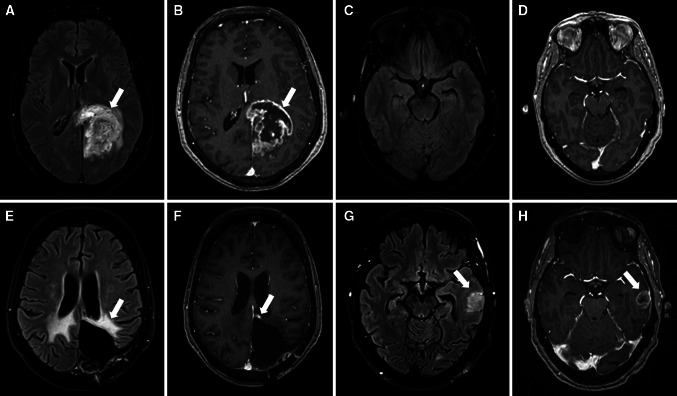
63 years-old female with GBM. MRI at diagnosis (*upper row*) and first recurrence (*lower row*). At diagnosis a left parieto-occipital mass was found, hyperintense in T2 FLAIR (*arrow* in a) and with irregular peripheral enhancement with central necrosis in T1Gd (*arrow* in b), with normal left temporal lobe (c and d). 13 months after surgery there was no evidence of recurrence at the surgical cavity borders with only treatment-related changes, with gliosis in T2 FLAIR (*arrow* in e) and a small stable area on enhancement in T1 Gad (*arrow* in f). Yet a new remote nodule appeared in the left temporal lobe, outside the radiation field (*arrows* in g and h), confirmed to be a GBM recurrence after surgery
